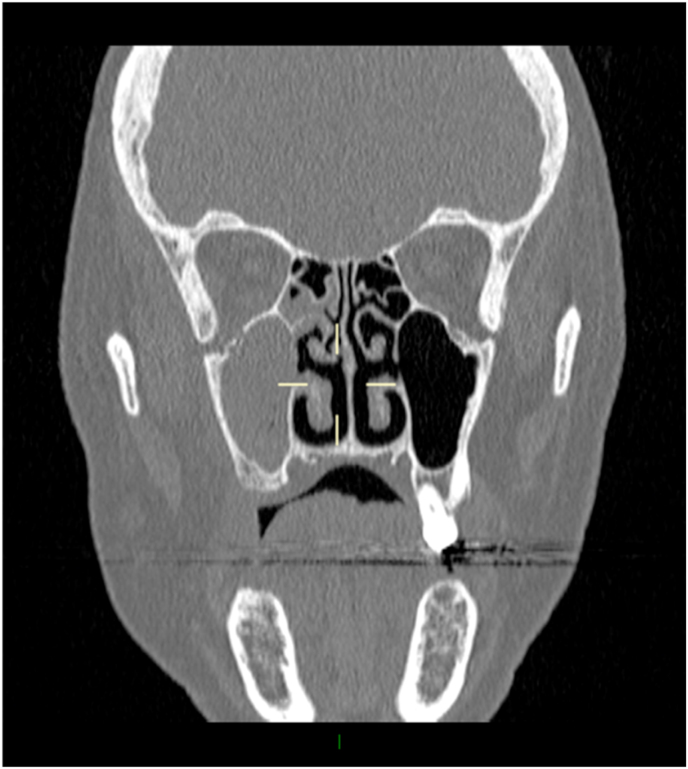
Evidence of right maxillary sinusitis with extension through the orbital floor (CT Sinus; coronal view).
